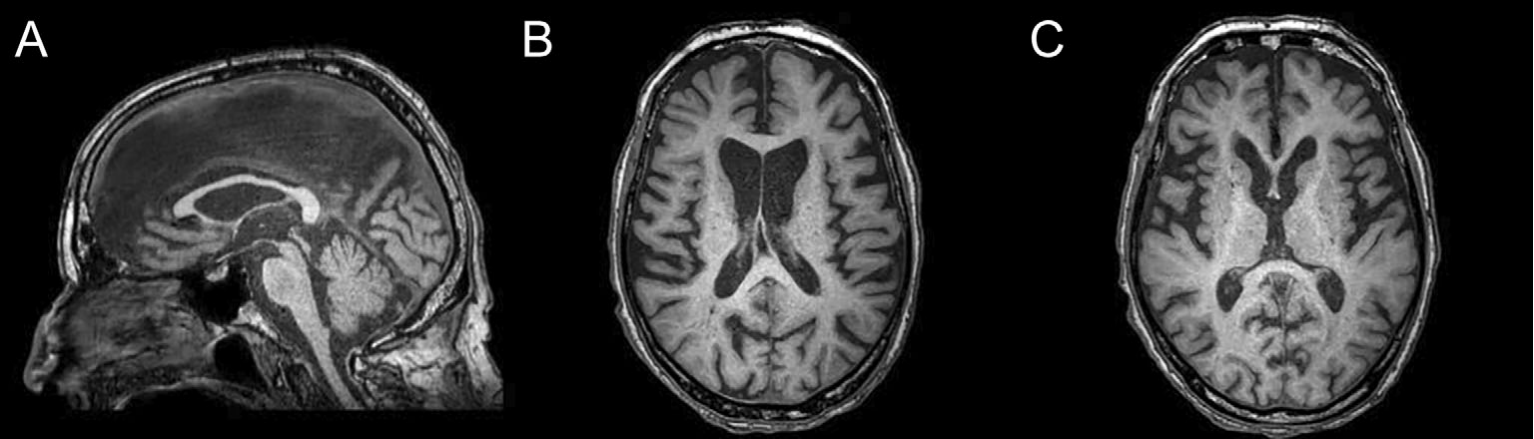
Brain MRI. Sagittal T1‐weighted image (A) showing midbrain atrophy with preserved pons corresponding to the hummingbird sign. Pons to midbrain area ratio (P/M = 6.01), third ventricle to frontal horns width ratio (3rdV/FH = 0.29) and Magnetic Resonance Parkinsonism Index 2.0 (MRPI 2.0 = 3.89) index indicated prominent midbrain atrophy, as observed in PSP‐RS. Axial T1‐weighted images showing bilateral frontal atrophy (B) without caudate atrophy (C).
